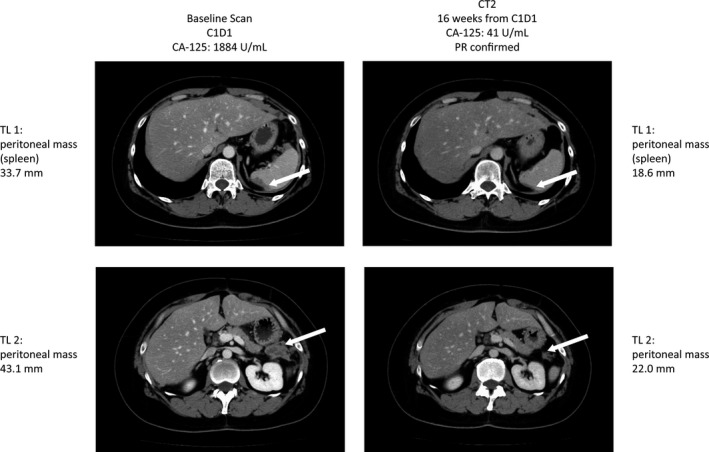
Computed tomography images showing partial response in a single patient to veliparib 200 mg BID. BID, twice‐daily; C1D1, day 1 of cycle 1; CA‐125, cancer antigen 125; CT, computed tomography; PR, partial response; TL, target lesion.
